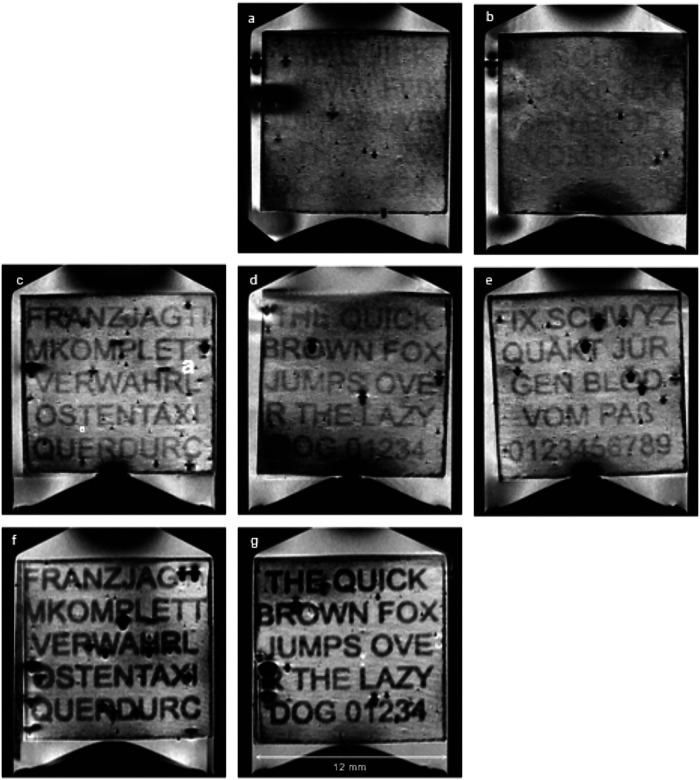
MRI-scanned book pages from a nine-page sample. Page reconstruction by cubic model (Eq. (3)). Toner layer thickness for the different number of layers: a, b first row *th*_2_ = 14 *µm* (two layers), c–e second row *th*_4_ = 28 *µm* (four layers), f, g third row *th*_8_ = 56 *µm* (eight layers). Each column corresponds to one distinct test sentence. The missing pages were omitted in the selection of MR-slabs and therefore could not be processed. Sheet numbers from top left to bottom right according to positioning (Fig. 9): 3 (missing),1,2; 6,4,5; 9,7,8 (missing). All images were automatically adjusted for white balance, which slightly improves contrast compared to Fig. 7c, f.
